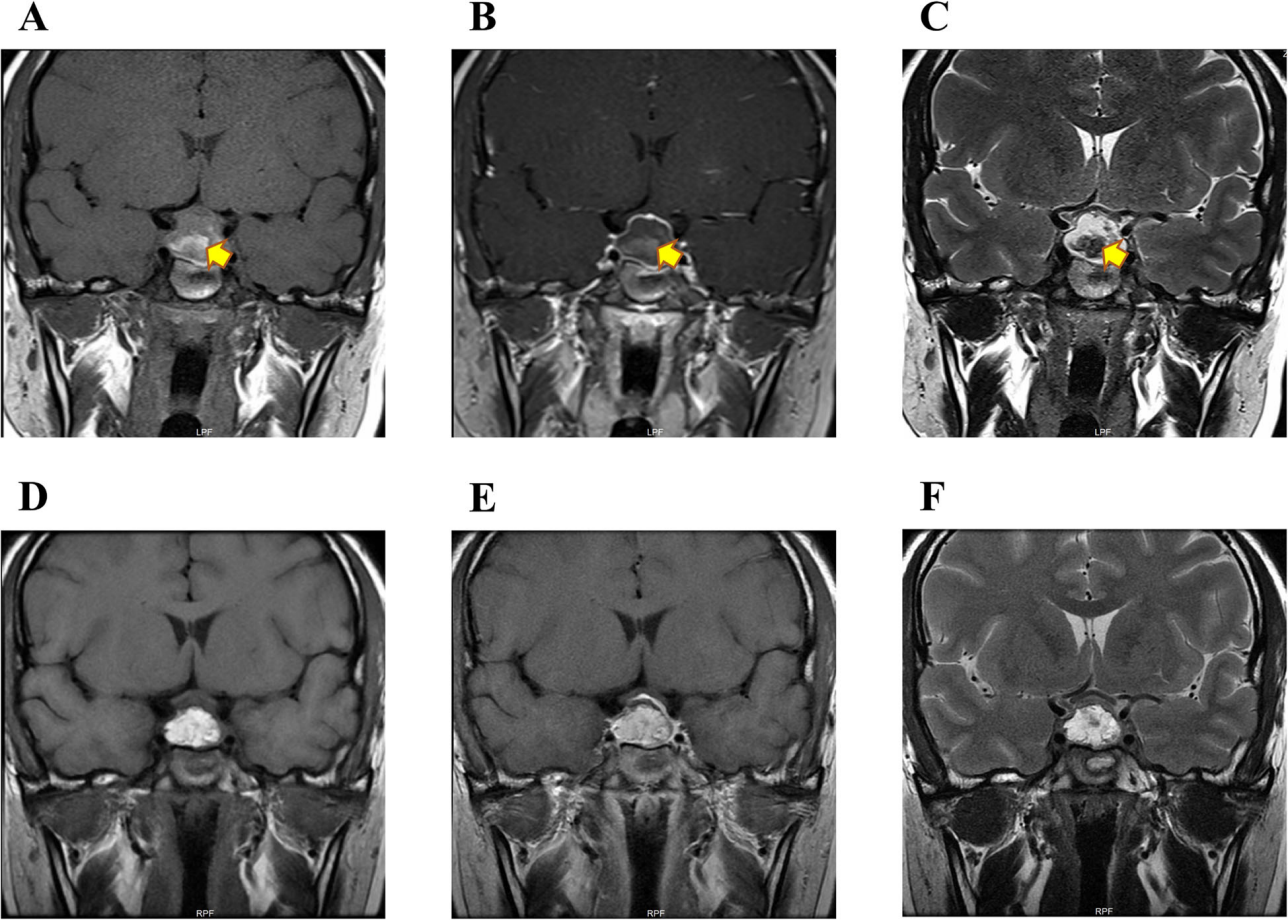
Coronal magnetic resonance imaging of pituitary in this patient. Before the surgery: pituitary apoplexy, with weakened signal in the posterior pituitary. A. T1; B. T1 + strengthen; C. T2. After the surgery: with stuffing added. D. T1; E. T1 + strengthen; F. T2
